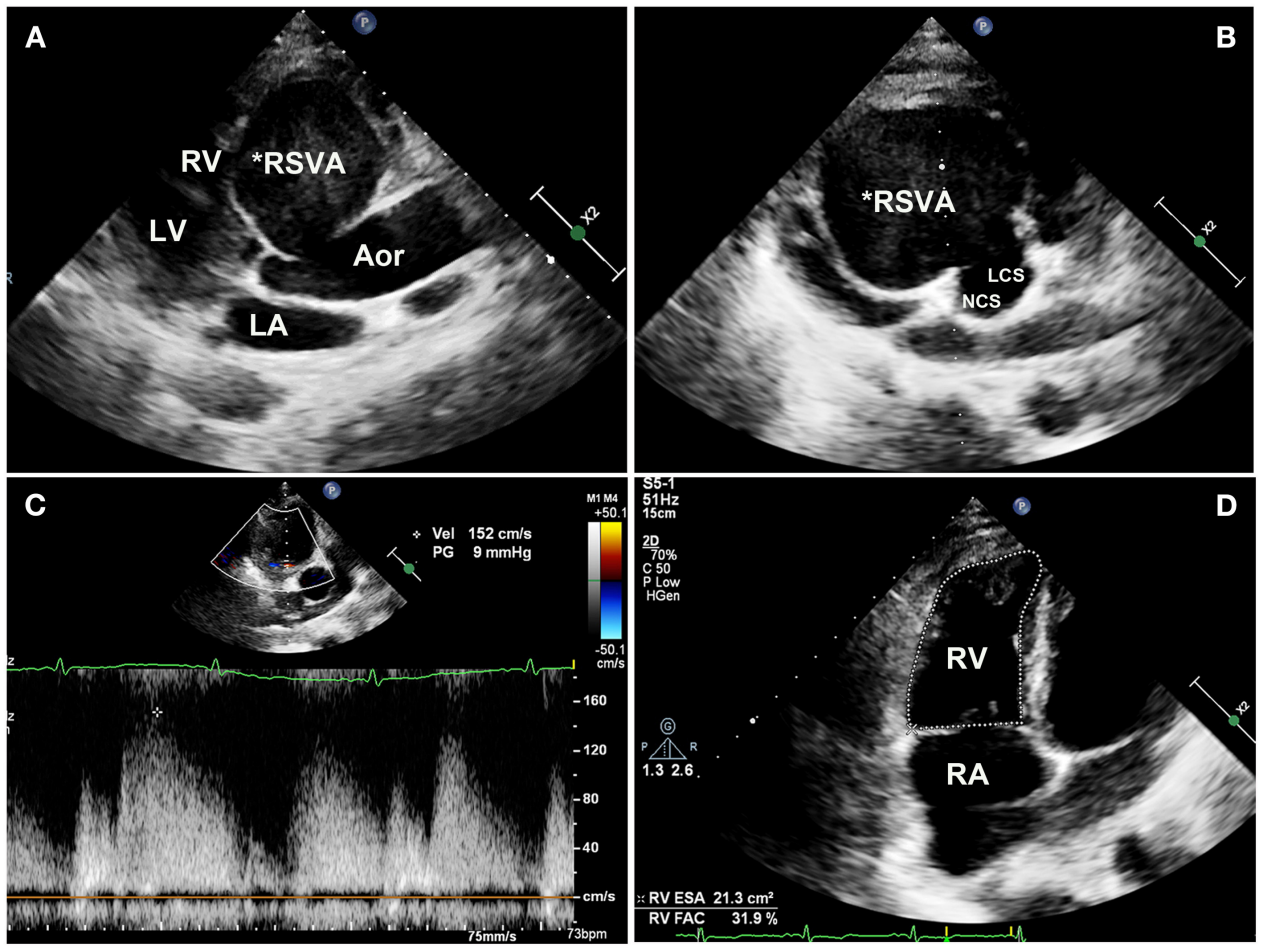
Transthoracic echocardiography images. (A) Transthoracic echocardiography showing a giant right sinus of Valsalva aneurysm in the long-axis view and (B) the aortic root. (C) The compressed RCA is displaced with an accelerating velocity of 150 cm/s. (D) The right ventricular systolic fraction is reduced (31.9% RVFAC). RSVA, right sinus of Valsalva aneurysm; RV, right ventricle; LV, left ventricle; LA, left atrium; Aor, aorta; NCS, noncoronary sinus; LCS, left coronary sinus; RA, right atrium; RVFAC, right ventricular fractional area change.
